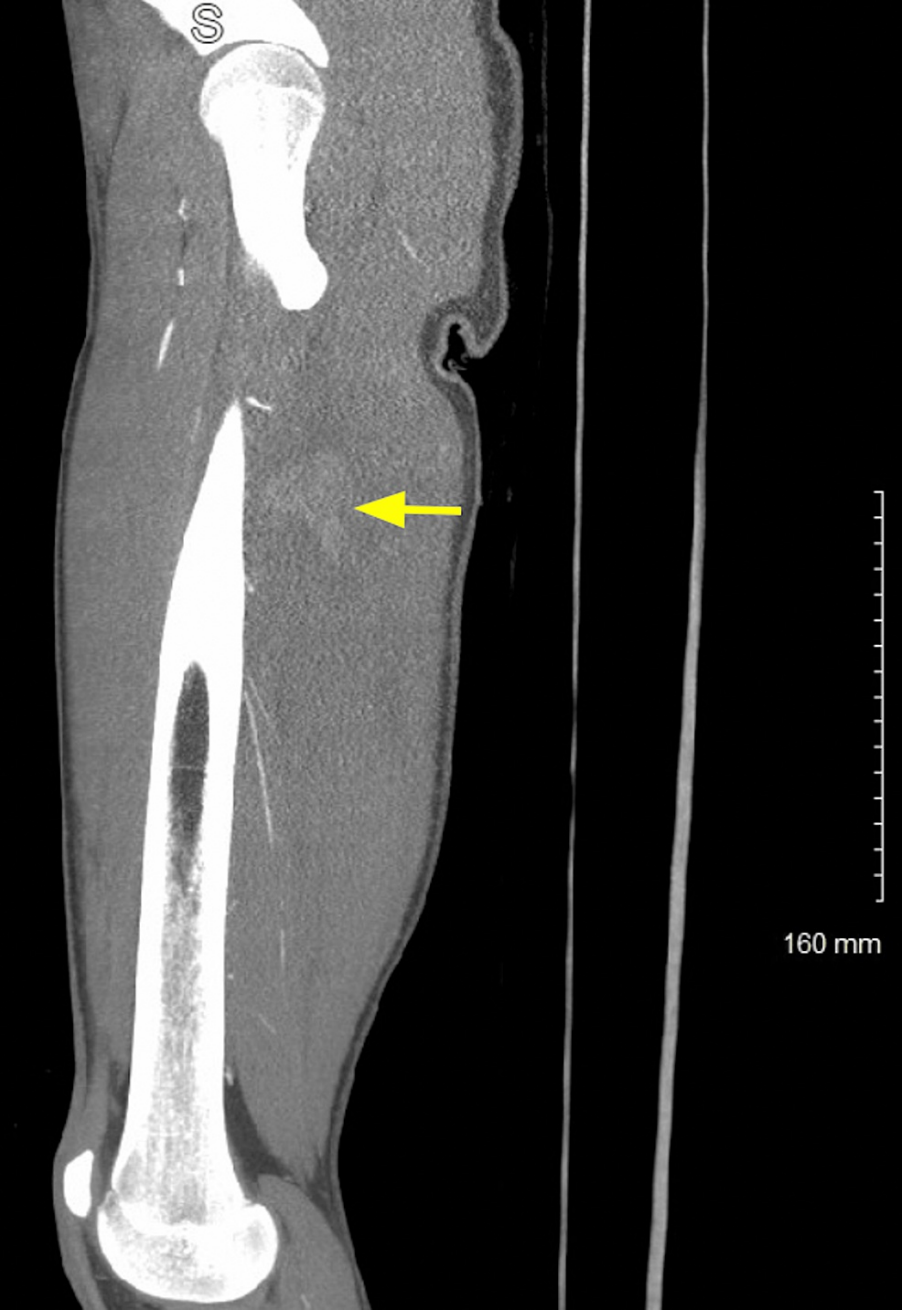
Sagittal CT angiogram The angiogram shows extravasation of contrast (arrow).
